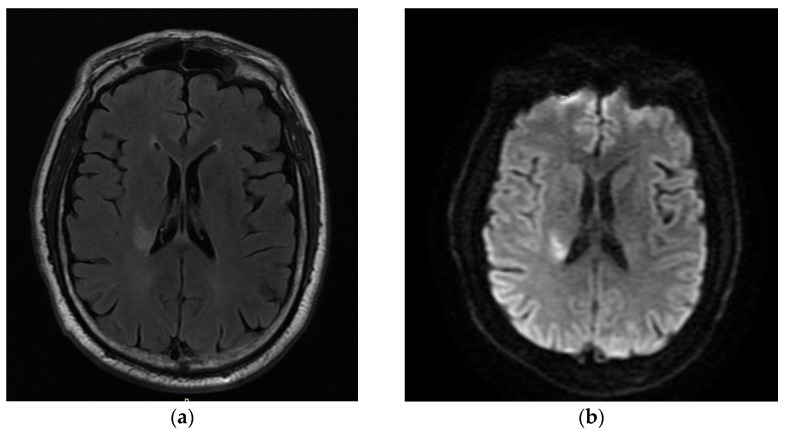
Brain MRI: axial flair (a) and DWI (b) manifesting right infarct extending from the right thalamus (posterior limb of the internal capsule) in the distribution of the right AChA.
